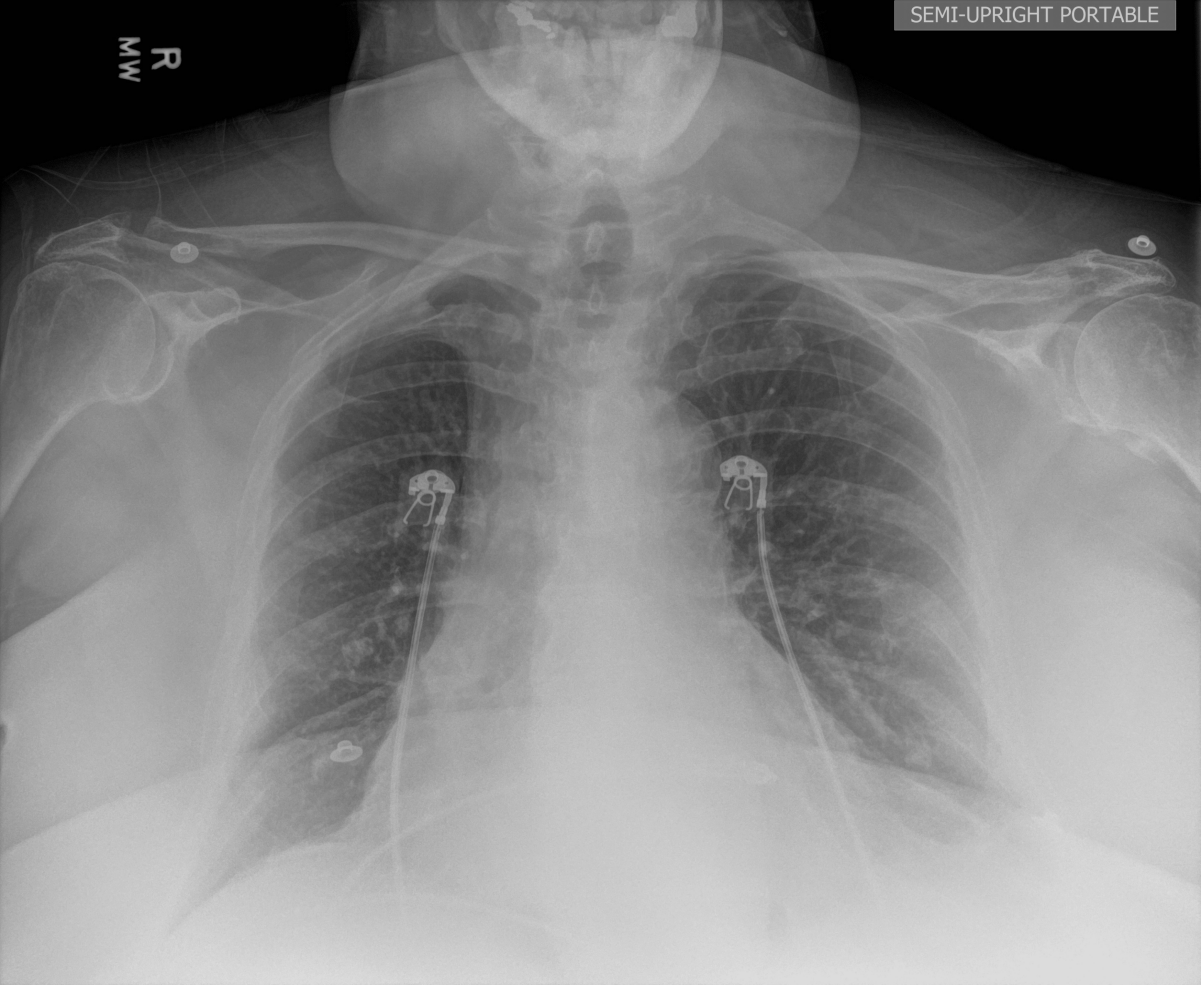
Upright chest X-ray showing a large cardiac silhouette consistent with heart failure, mild vascular congestion, left basilar atelectatic changes, and a flattened diaphragm
